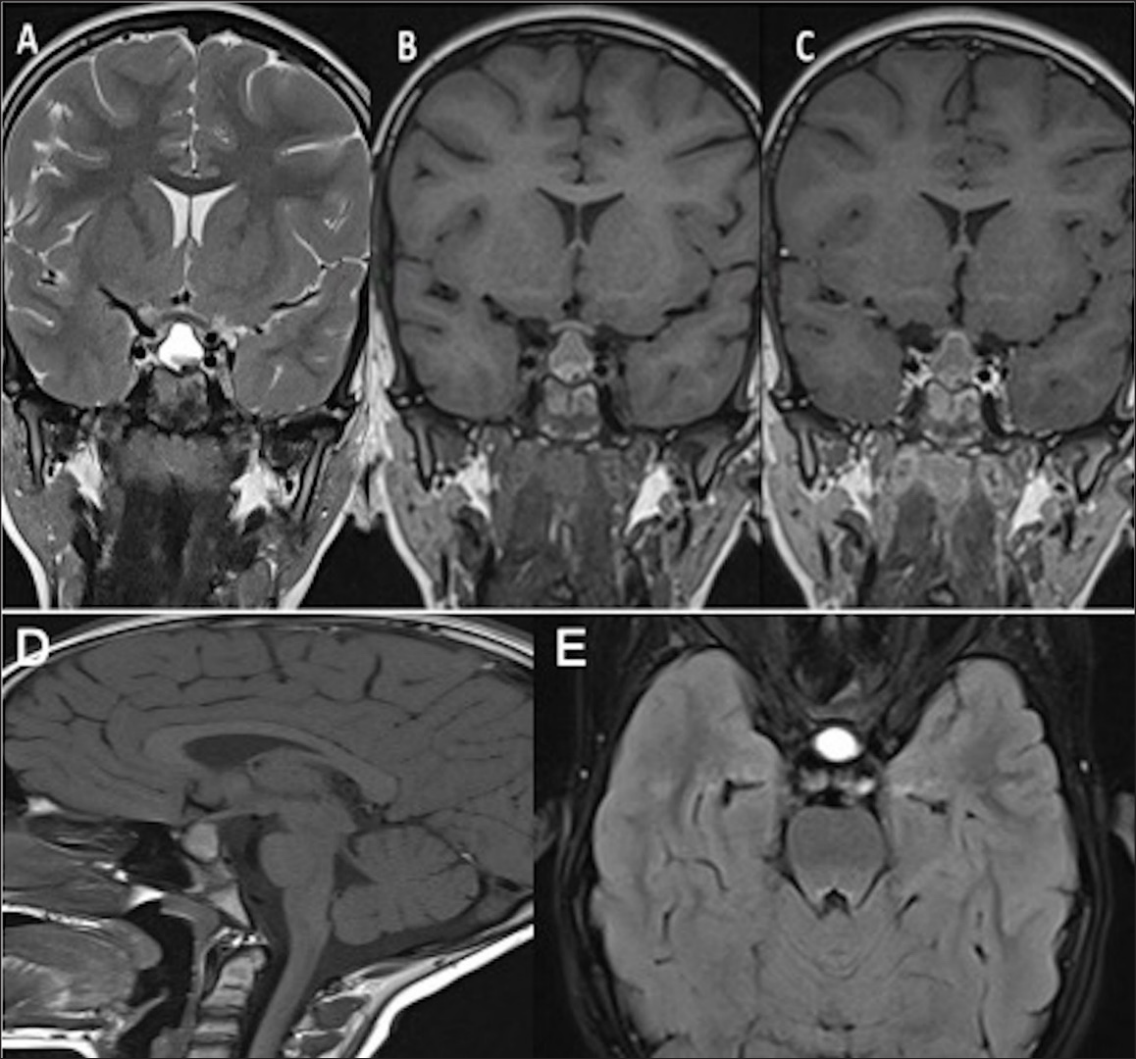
Coronal T2WI (A), T1WI (B), post-contrast T1WI (C), sagittal T1WI (D) and axial FLAIR (E) images demonstrate a sellar and suprasellar lesion with heterogeneous content in the lower part of the lesion and peripheric enhancement.
